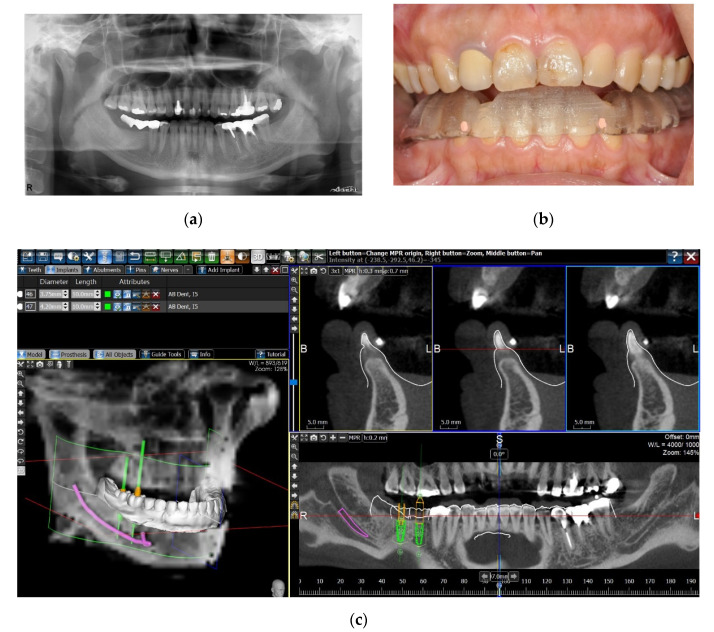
Matching the digital dental cast and the CBCT scan by registration of fiducial markers. (a) A patient with missing right mandibular first and second molars. Because of the streaking artifacts from the radiopaque crowns of bilateral posterior area on the CBCT scan, a direct superimposition with the digital dental cast was challenging. (b) On the basis of the digital dental cast, an SLA radiographic template with gutta percha fiducial markers was fabricated. The patient took a CBCT scan with the radiographic template in the mouth. (c) The image of the fiducial markers could be identified on both the digital dental cast and the CBCT scan, and the two digital images could be superimposed by matching the fiducial markers.
